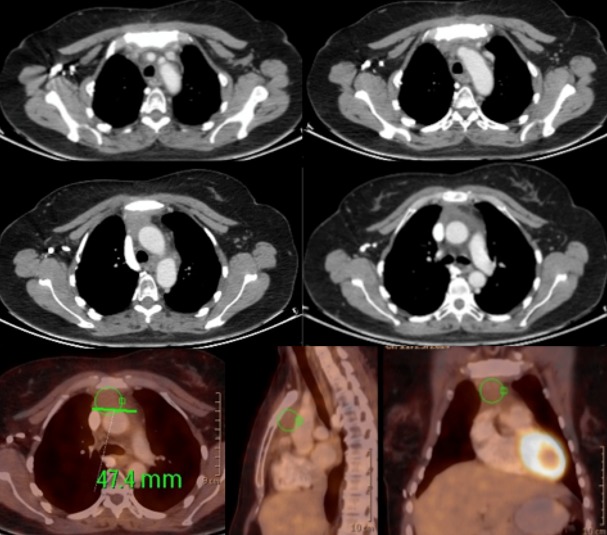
Chest CT: downsizing of the mediastinal; remains in close contact with vascular structures without a clear separation line with no sternal involvement; PET-TC: not FDG accumulation in remaining tissue
